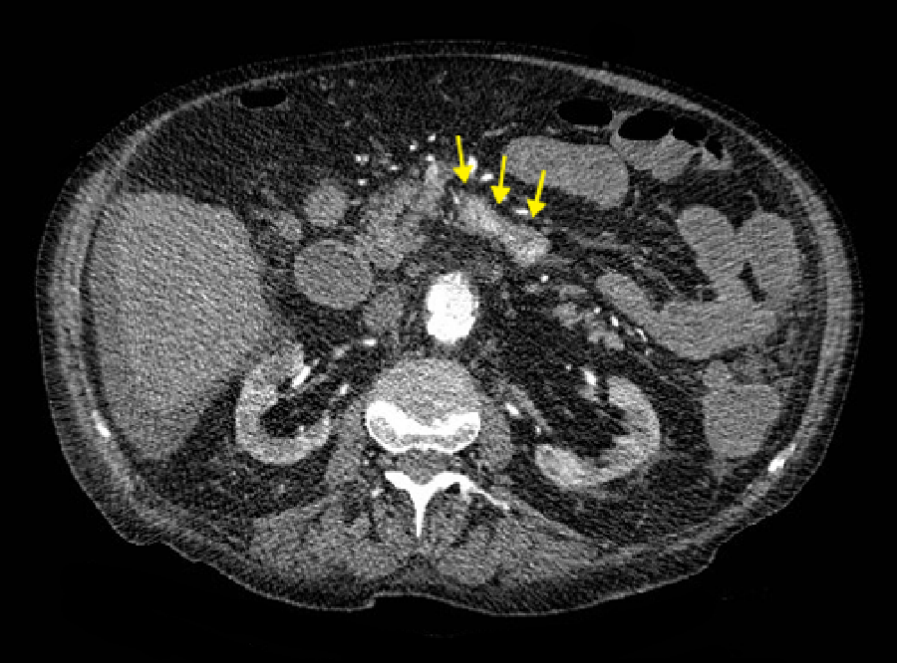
Abnormal Contrast in the Duodenum Axial contrast-enhanced (IV only) CT image at the level of the kidneys showing the abnormal presence of contrast in the 3rd part of the duodenum (arrows).
